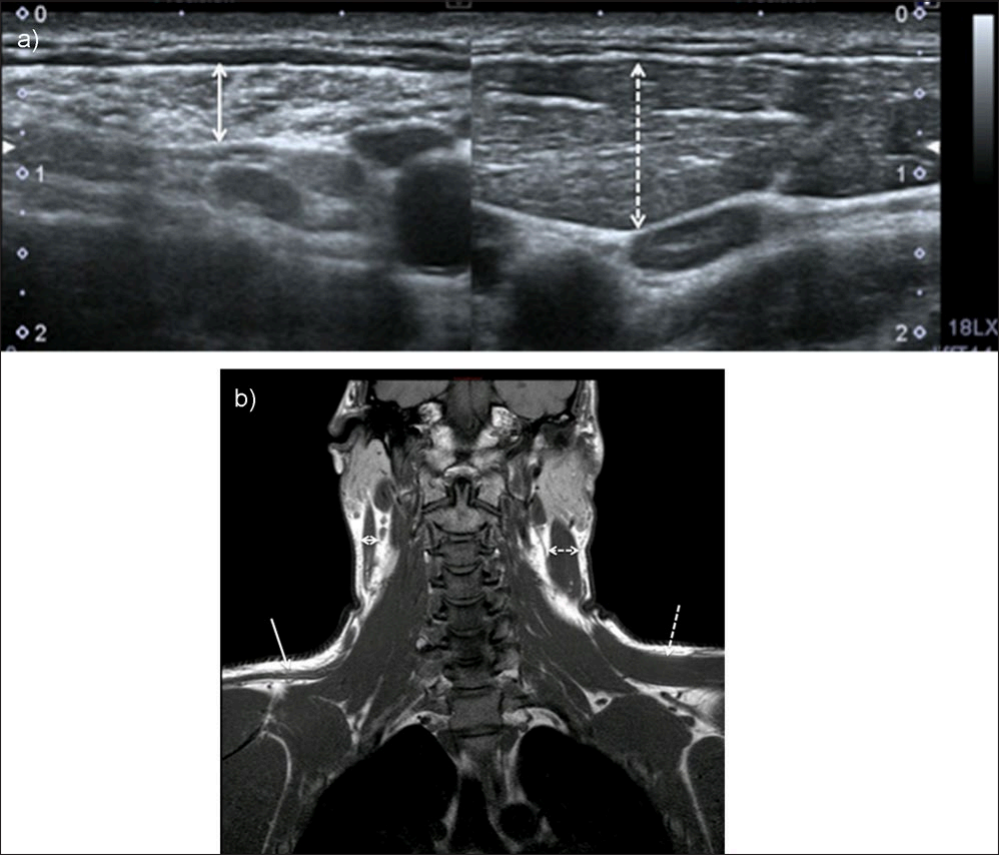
Chronic neuropathy of spinal accessory nerve. Ultrasound showed atrophy of sternocleidomastoid muscle (double solid arrow) compared to normal side (doubled dashed arrow) (5a = FIGURE 13 uploaded online manuscript), confirmed by coronal T1-weighted MRI showing both atrophy of trapezus (solid arrow) and sternocleidomastoid muscles compared to normal side (dashed arrow) (5b = FIGURE 14 uploaded online manuscript).
